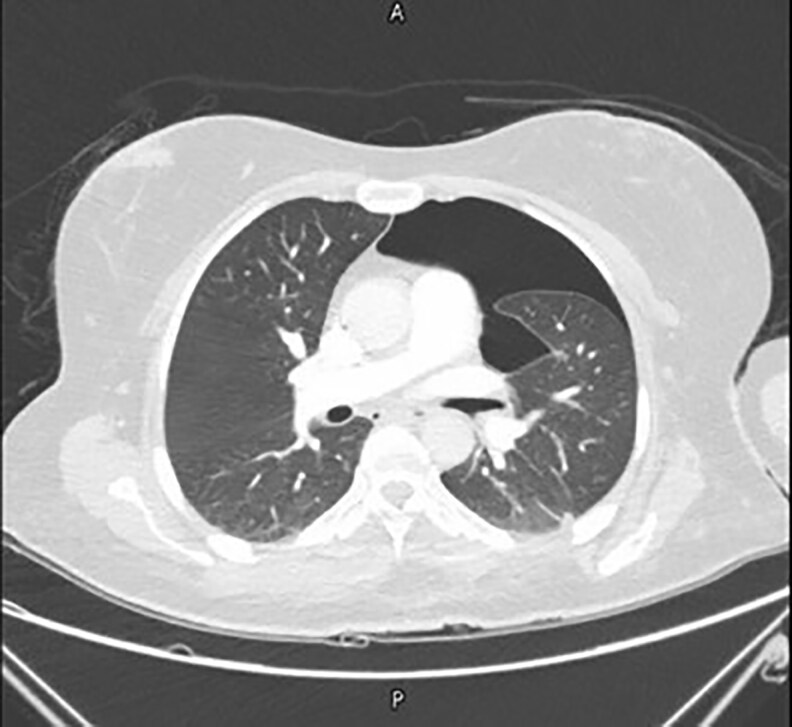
Computed tomography scan axial view showing a collapsed left lung in a 48-year-old female patient, confirming moderate pneumothorax.
